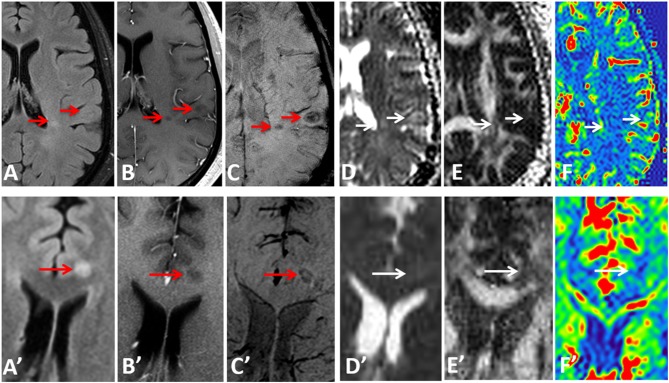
Representative images of Type-1 lesions in two MS patients (top row from a 36-year-old male patient and bottom row from a 37-year-old female patient) include FLAIR (A,A′), Gd-enhanced T1-weighted (B,B′), and SWI (C,C′), as well as parameter maps of MD (D,D′), FA (E,E′), and CBF (F,F′). The hypointense lesions on SWI (arrows) are associated with a less significant change in diffusion and perfusion measurements, as compared perilesional NAWM.
